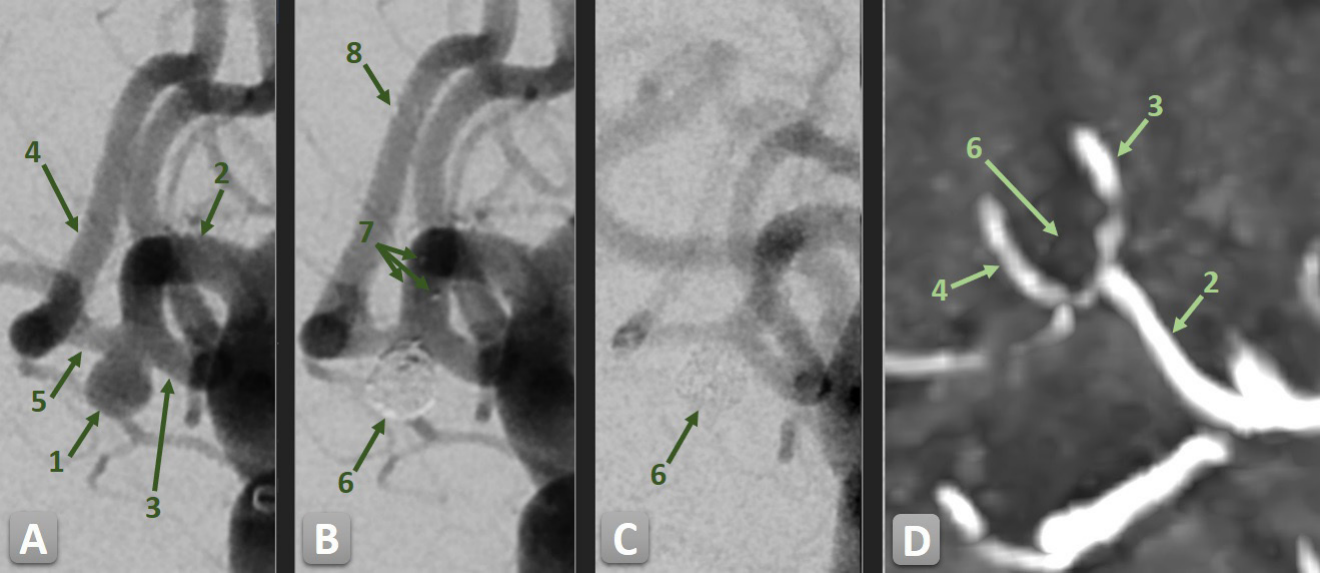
DSA of a relatively wide-necked unruptured aneurysm of the anterior communicating artery (AComA) treated with stent-assisted coiling (SAC) using a single pEGASUS-HPC stent, providing sufficient neck coverage. (1) AComA aneurysm. (2) A1 segment of the left anterior cerebral artery (ACA). (3) A2 segment of the left ACA. (4) A2 segment of the right ACA. (5) AComA. (6) Coiled aneurysm. (7) Proximal stent markers in the A1 segment of the left ACA. (8) Distal stent markers in the A2 segment of the right ACA. (A) Pre-treatment 2D angiogram showing the aneurysm and adjacent vascular anatomy. (B) Post-treatment 2D angiogram following stent deployment and coil embolization showing proximal and distal stent markers. A small residual perfusion at the aneurysm neck is visible. (C) Angiographic follow-up at 3 months showing progressive, now complete occlusion of the aneurysm sac. The weak contrast opacification is due to the proximal catheter position. (D) Last MRI follow-up (time-of-flight) at 2 years confirming persistent and complete aneurysm occlusion.
